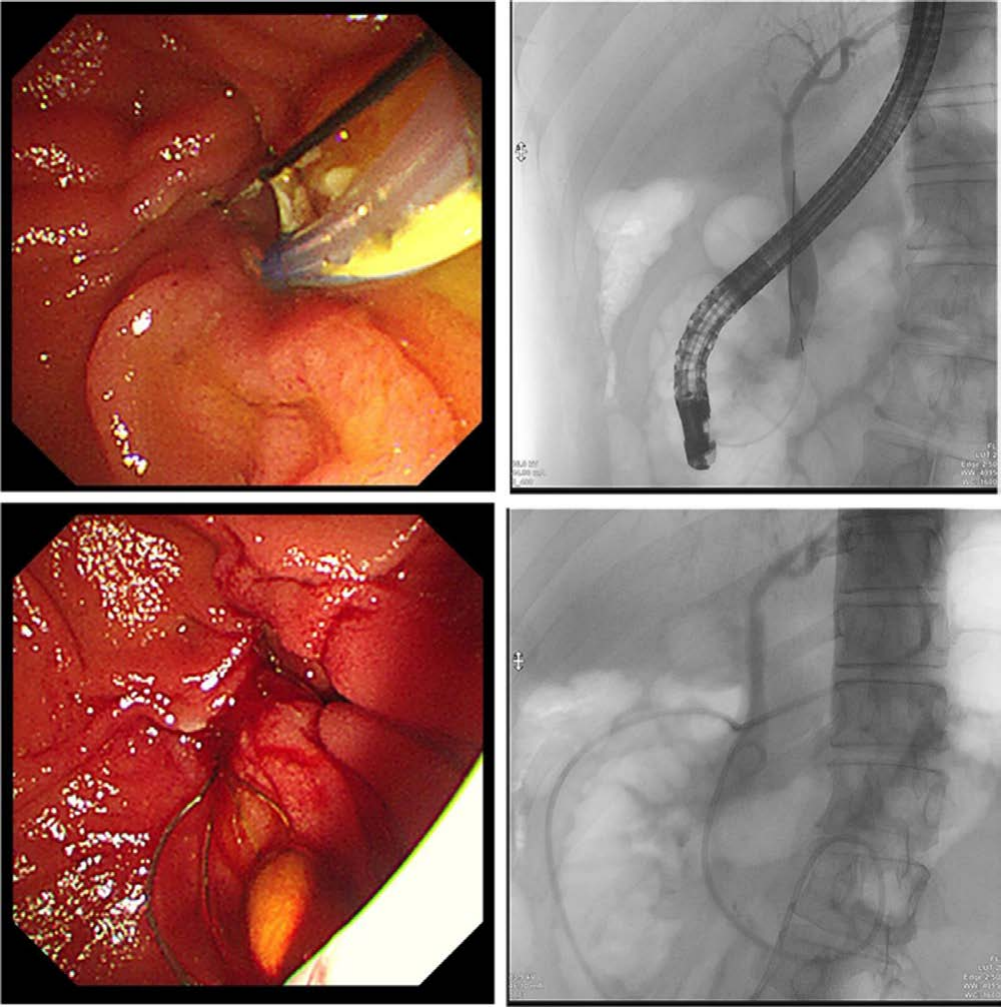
ERCP + EST + X-ray CBD stone + basket stone retrieval + balloon-sweeping ENBD. CBD = common bile duct, ENBD = endoscopic nasobiliary drainage, ERCP = endoscopic retrograde cholangiopancreatography, EST = endoscopic sphincterotomy.
